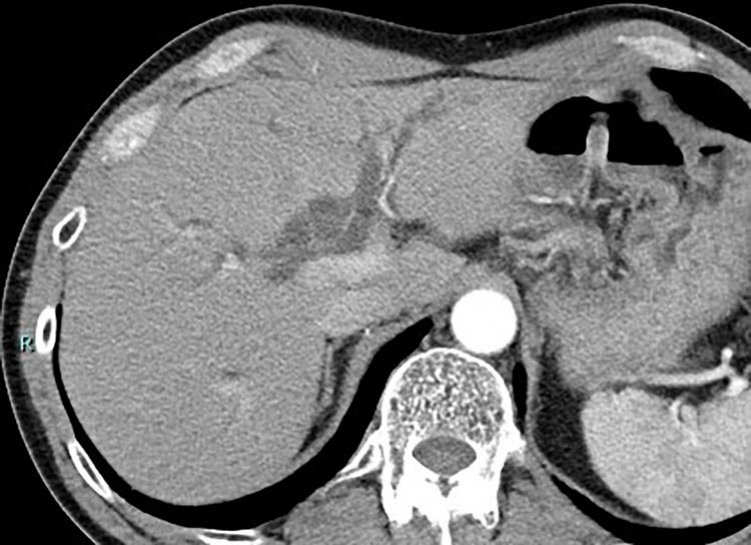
CT scan showed severe dilation of intrahepatic bile duct.
